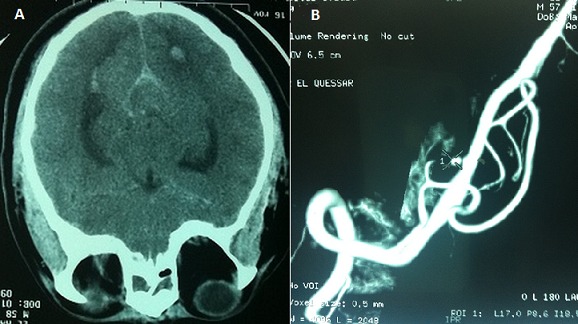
(A) Brain CT: ventricular flood with spontaneous hyperdensity of sulci and sylvian valleys; (B): magnetic resonance angiography (MRA): aneurysm of the initial segment of posterior inferior cerebellar artery (PICA)
